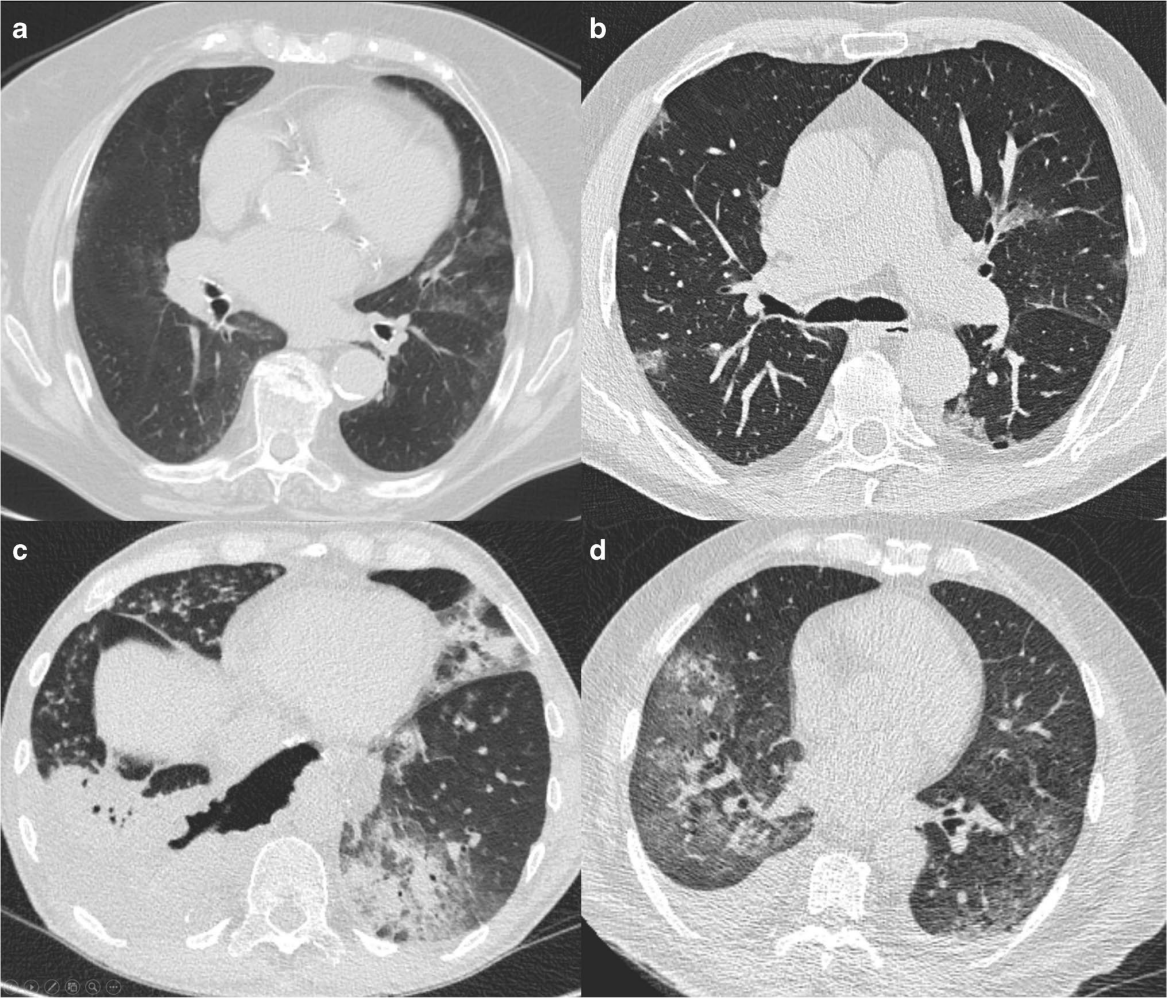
a–d Examples in which all AI solutions categorized the CT studies from 2018 as COVID-19 suspect
